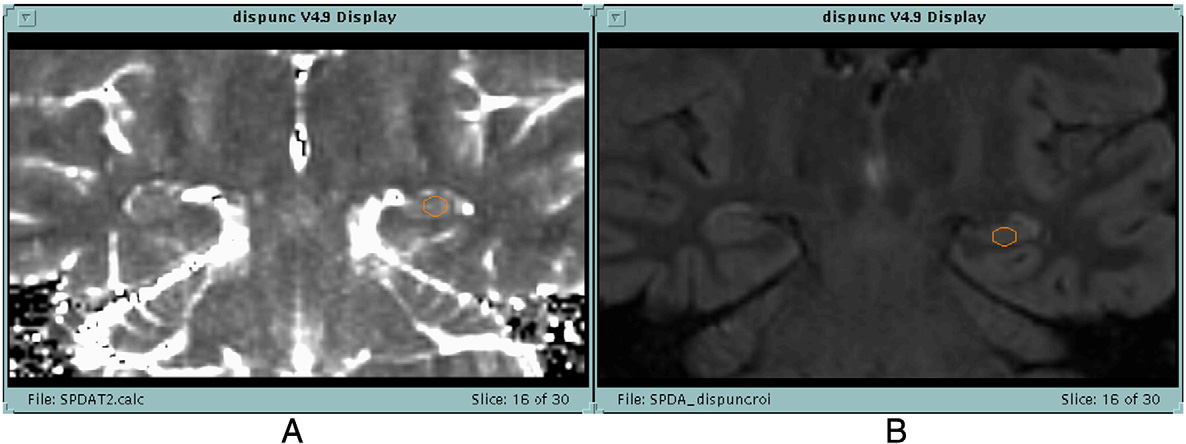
Placement of ovoid ROIs on hippocampal slices of T2 map A and T2 FLAIR (B) images (Bartlett et al., 2007).
